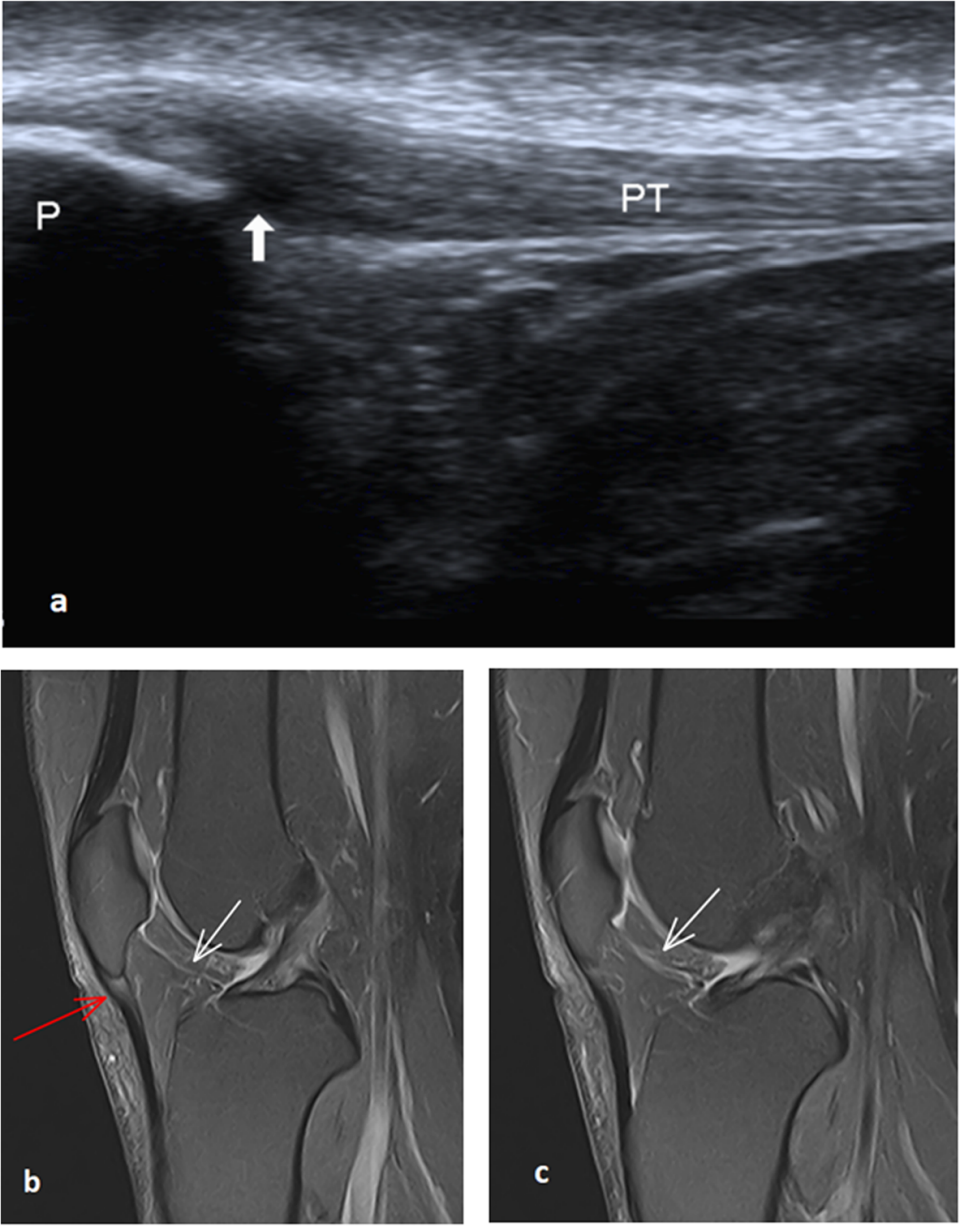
A 45-year-old female with right patellar tendinopathy and infrapatellar plica. a Long axis ultrasound image through the patellar tendon shows a focal thickening of the proximal part of the patellar tendon with a localized hypoechoic area sparing the anterior fibers. (White thick arrow). b and c Two subsequent sagittal PDFS MRI images through intercondylar notch show focal proximal tendon thickening and increased signal intensity (red arrow) sparing the anterior tendon fibers. Infrapatellar plica (white arrow) appears as a curvilinear high signal passing through Hoffa’s fat pad (not detected by ultrasound). P, patella; PT, patellar tendon
